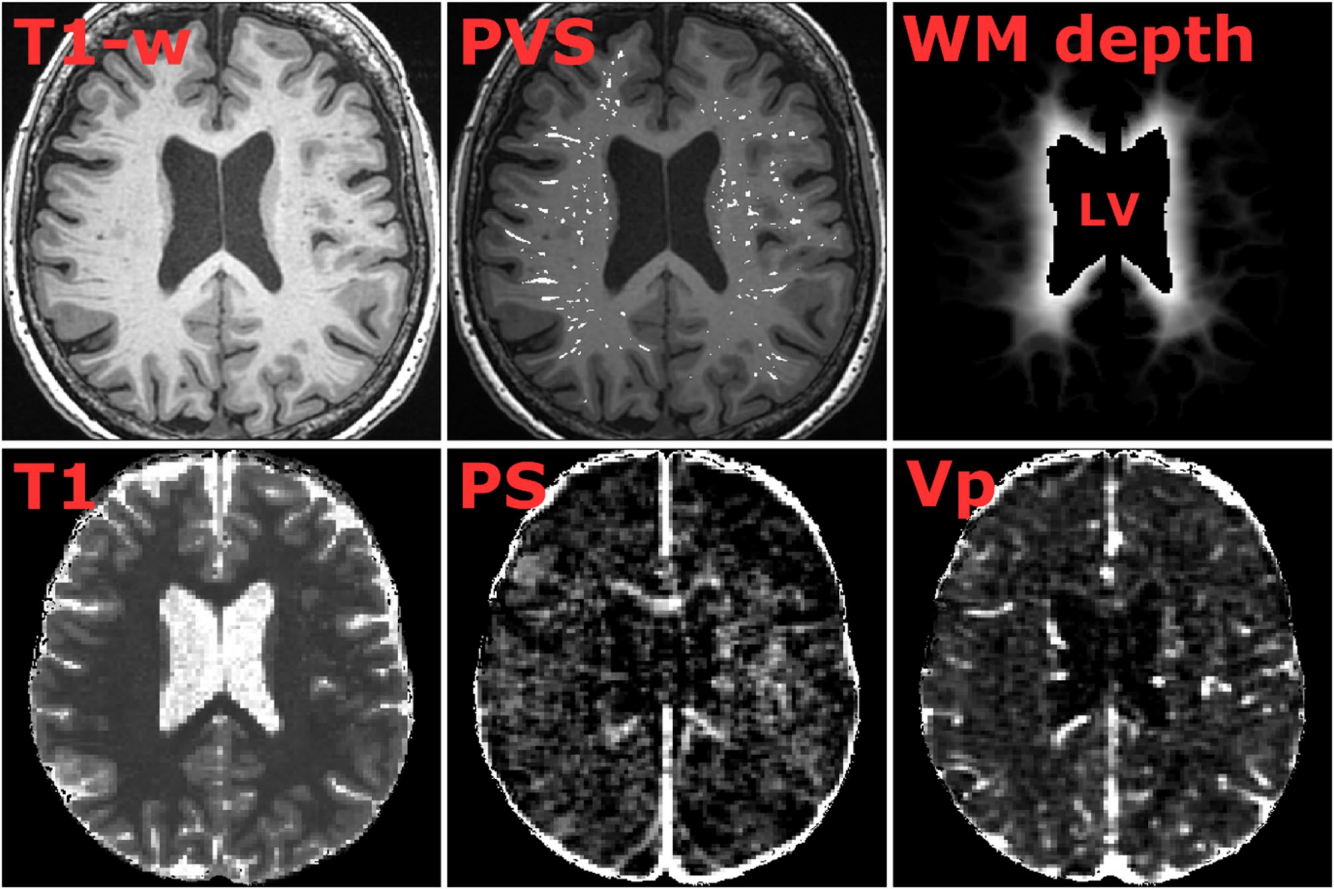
Anatomical T1-weighted MRI, segmented perivascular spaces (PVS), normalized cortex-ventricular white matter (WM) depth, baseline T1 map, permeability-surface area product (PS), and fractional plasma volume ($$\:{v}_{p}$$) for an example participant. Quantitative visualizations range from zero to a maximum of 5s (T1), 2.5$$\:\cdot\:$$10^−3^$$\:\cdot\:$$ min^− [1]^ (PS), and 5% ($$\:{v}_{p}$$). Note that ROI-averaged concentration curves were used to estimate PS and $$\:{v}_{p}$$ for all statistical analyses, whereas these parameter maps were intended for visualization only
